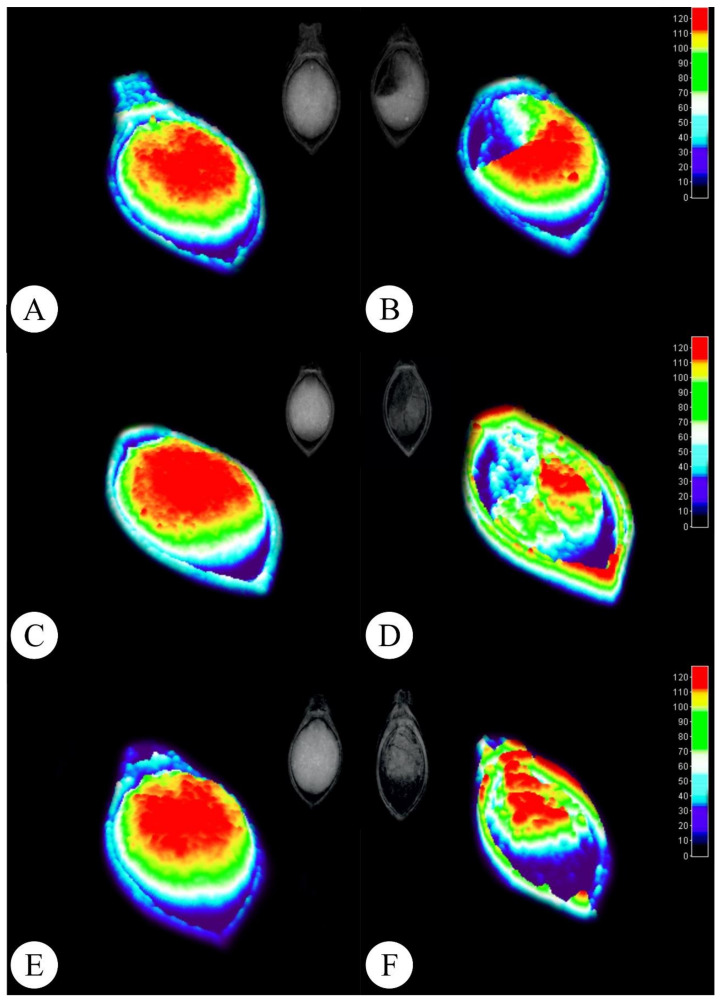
Radiographic *Brachiaria brizantha* seed images and their representations in 3D. (A,B) Marandu cv. (C,D) Piatã cv. (E,F) Xaraés cv. Left column: higher tissue density seeds. Right column: lower tissue density seeds.
